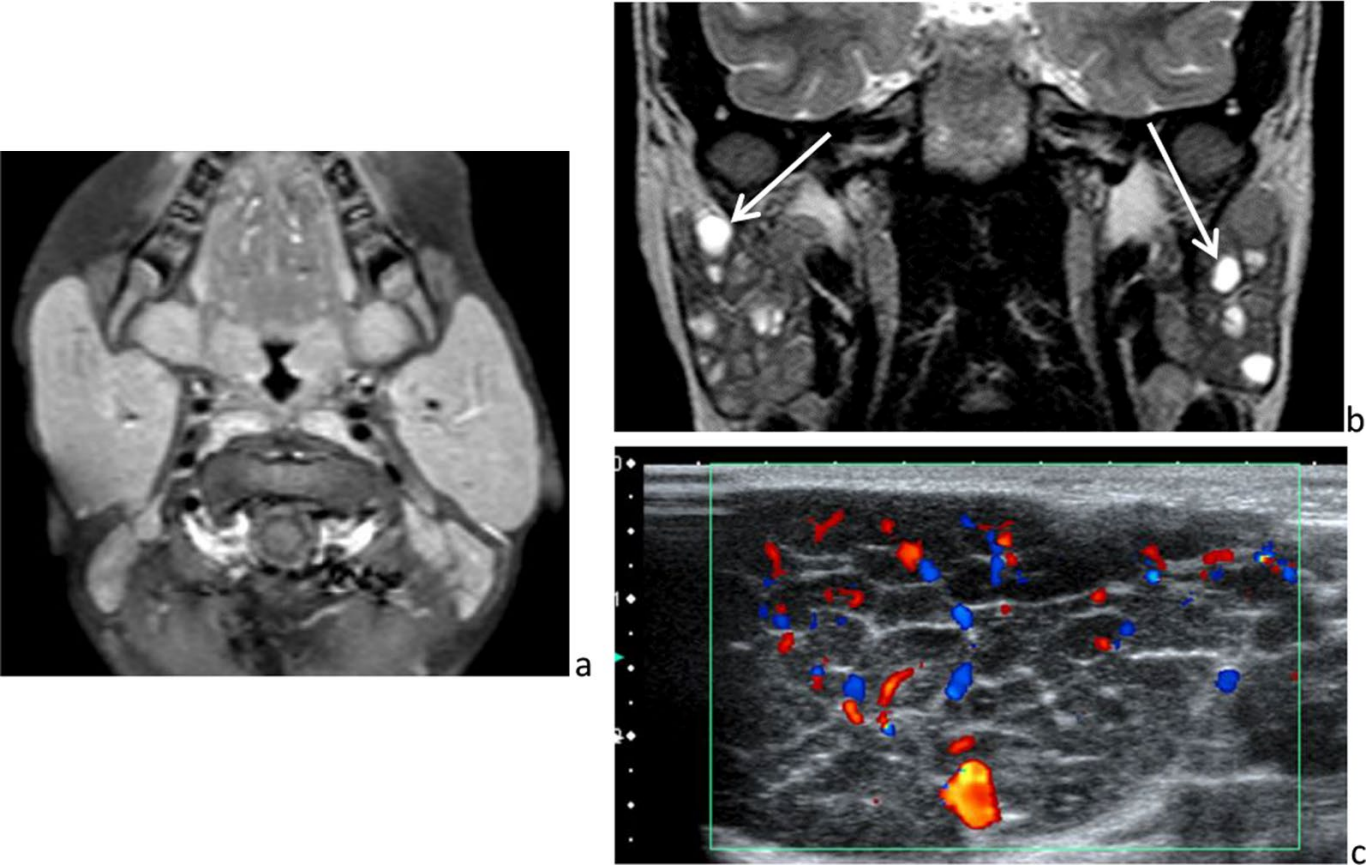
HIV-related disorders in 3 different patients. Diffuse homogeneous parotid enlargement on axial *T*_1_-weighted image, after intravenous administration of gadolinium chelate (a), lympho-epithelial cysts on frontal *T*_2_-weighted image (b) and benign lympho-epithelial lesions, hypoechoic on ultrasonography (c)
